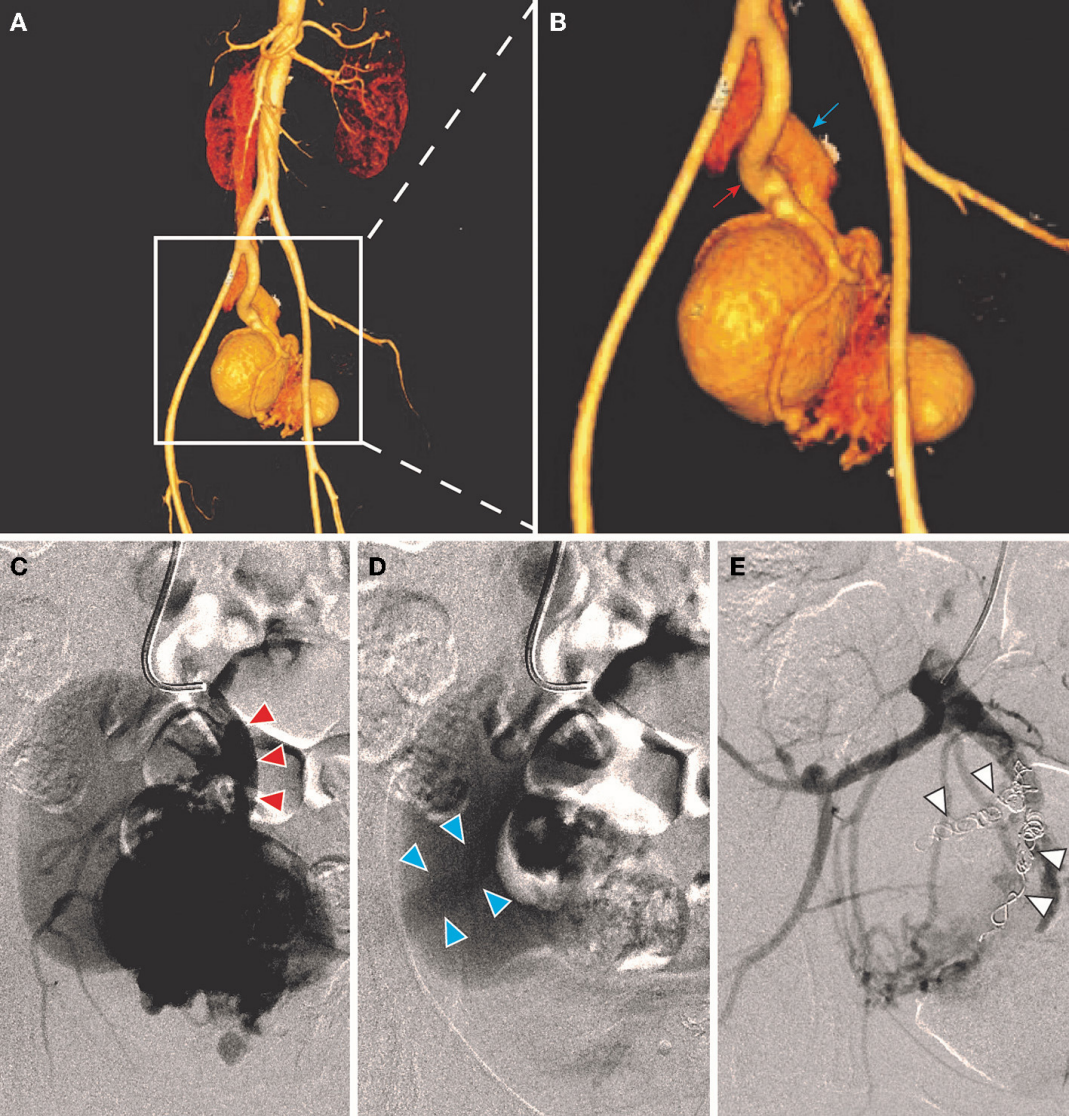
A 36-year-old female (case 2) was diagnosed as IAVFs and treated with transarterial embolization. (A,B) CTA showed communications between right internal artery (red arrow) and vein (blue arrows). (C,D) Transarterial angiography showed inflow arteries (red triangle arrows) and outflow veins (blue triangle arrows). (E). Coil embolization of branches of right internal iliac artery (white triangle arrows).
